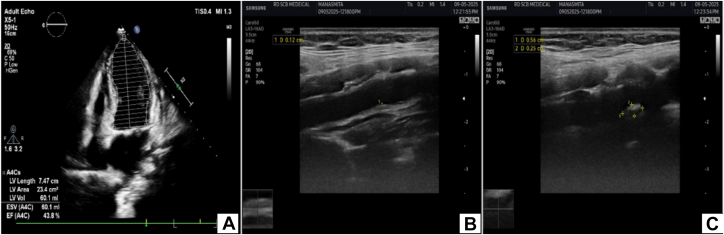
Carotid Intima-Media Thickness of Case 1 (A) Echocardiography showing an ejection fraction of 42%. (B) Ultrasound Doppler showing increased CIMT in the right common carotid artery. (C) Ultrasound Doppler showing calcified plaque of 5 × 3 mm at the bulb of the left common carotid artery. CIMT = carotid intima-media thickness.
